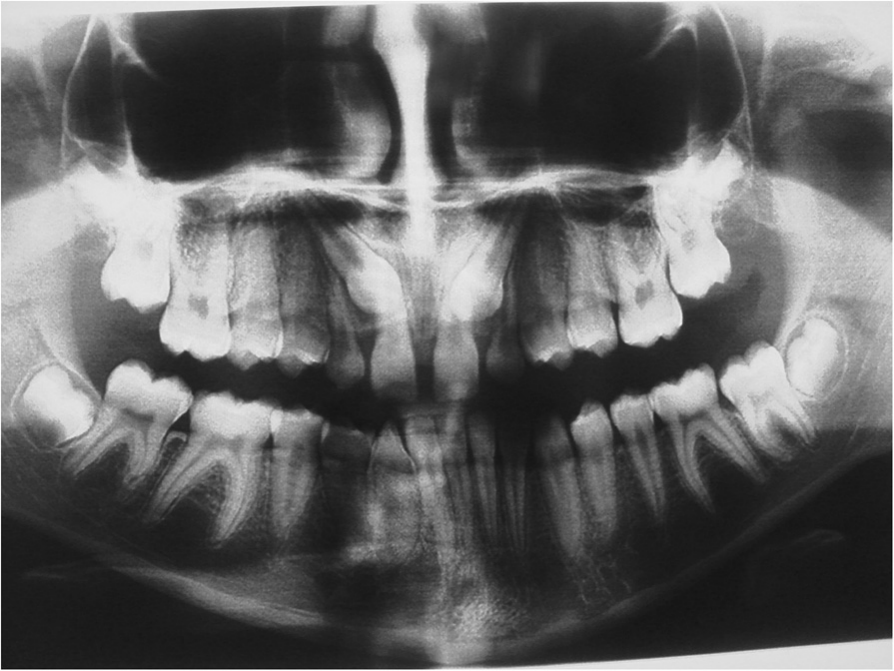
Orthopantomography with agenesis of 1.2 and 2.2.
